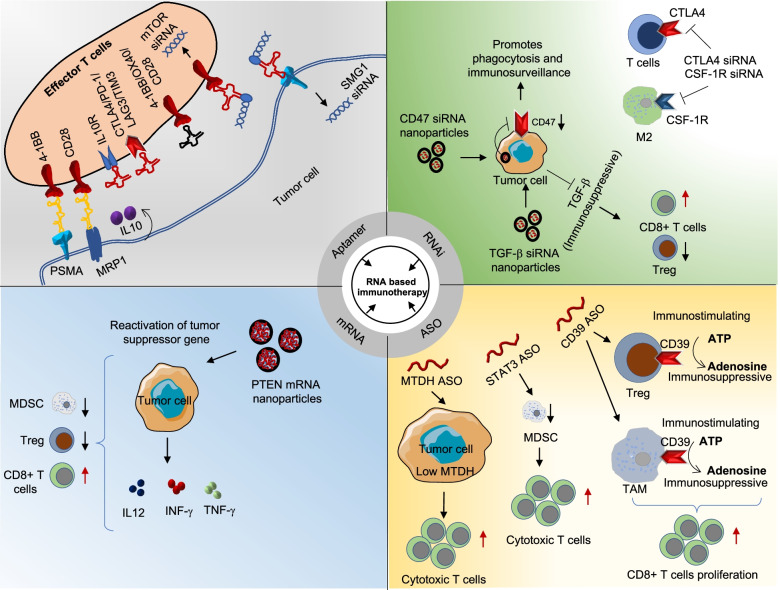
RNA-based modulation of the TIME and immunotherapy. Effector T cells, which play an important role in the antitumor immune response, can be targeted by different kinds of in vitro-transcribed RNA aptamers such as bispecific, antagonist, and chimeric. The interaction of these aptamers helps to prolong survival and inhibits T cell exhaustion that is linked with upregulation of antitumor immunity. Blocking immunosuppression-associated molecules using ASOs is another approach for cancer immunotherapy. Targeting immunosuppressor and immune-evading molecules such as CD47, TGF-β, CTLA-4, and CSF-1R by delivering their specific siRNAs in the form of NPs could lead to strong antitumor immune response. CD39 ASO downregulates CD39 expression on Tregs and TAMs, which are responsible for conversion of immunostimulating ATP into the immunosuppressive molecule adenosine. Modulating the function of MDSCs using STAT3 ASO results in the recruitment of higher levels of cytotoxic T cells. Targeting MTDH in the tumor cells with ASOs promotes antitumor immune response. Activating the tumor suppressor genes such as PTEN using PTEN mRNA-loaded NPs helps to inhibit the tumor growth by downregulating MDSCs and Tregs and activating CD8 + T cells. TGF-β, transforming growth factor-β; TNF-α, tumor necrosis factor α; INF-γ, Interferon gamma; IL, interleukin; IL-10R, interleukin-10 receptor; PSMA, prostate-specific membrane; LAG3, lymphocyte activation gene 3 protein; PD-1, programmed cell death protein 1; TIM3, T cell immunoglobulin mucin receptor 3; CSF-1R, colony-stimulating factor 1 receptor; ATP, adenosine triphosphate
